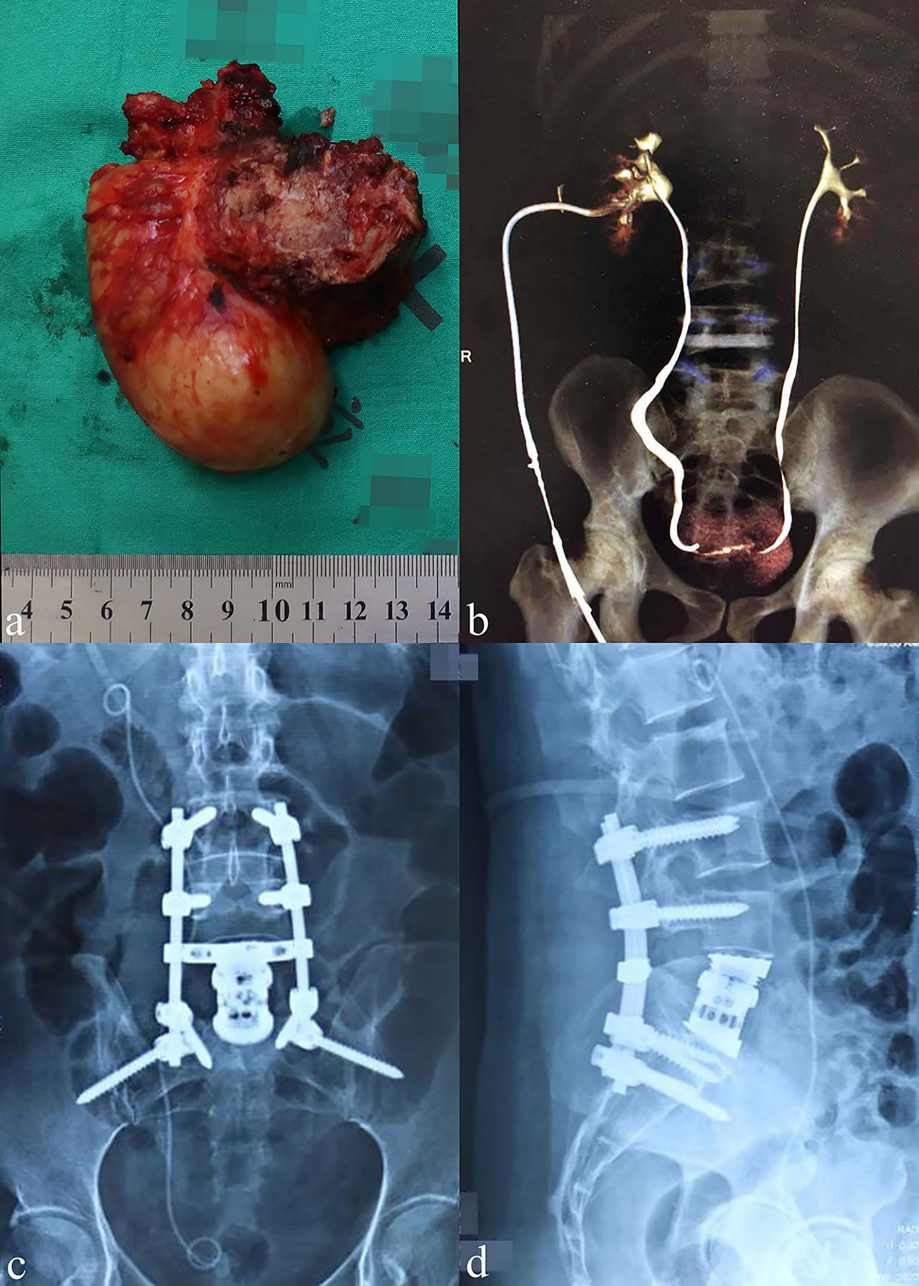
General view of the resected tumor and postoperative examinations. (A) General view showing a representative resected tumor (18 × 9 cm) in an *en bloc* fashion. (B) The postoperative intravenous pyelography indicated a continuous urinary flow on both sides of the ureters. (C, D) The anteroposterior and lateral X-ray of two patients at 24 months post-operatively.
